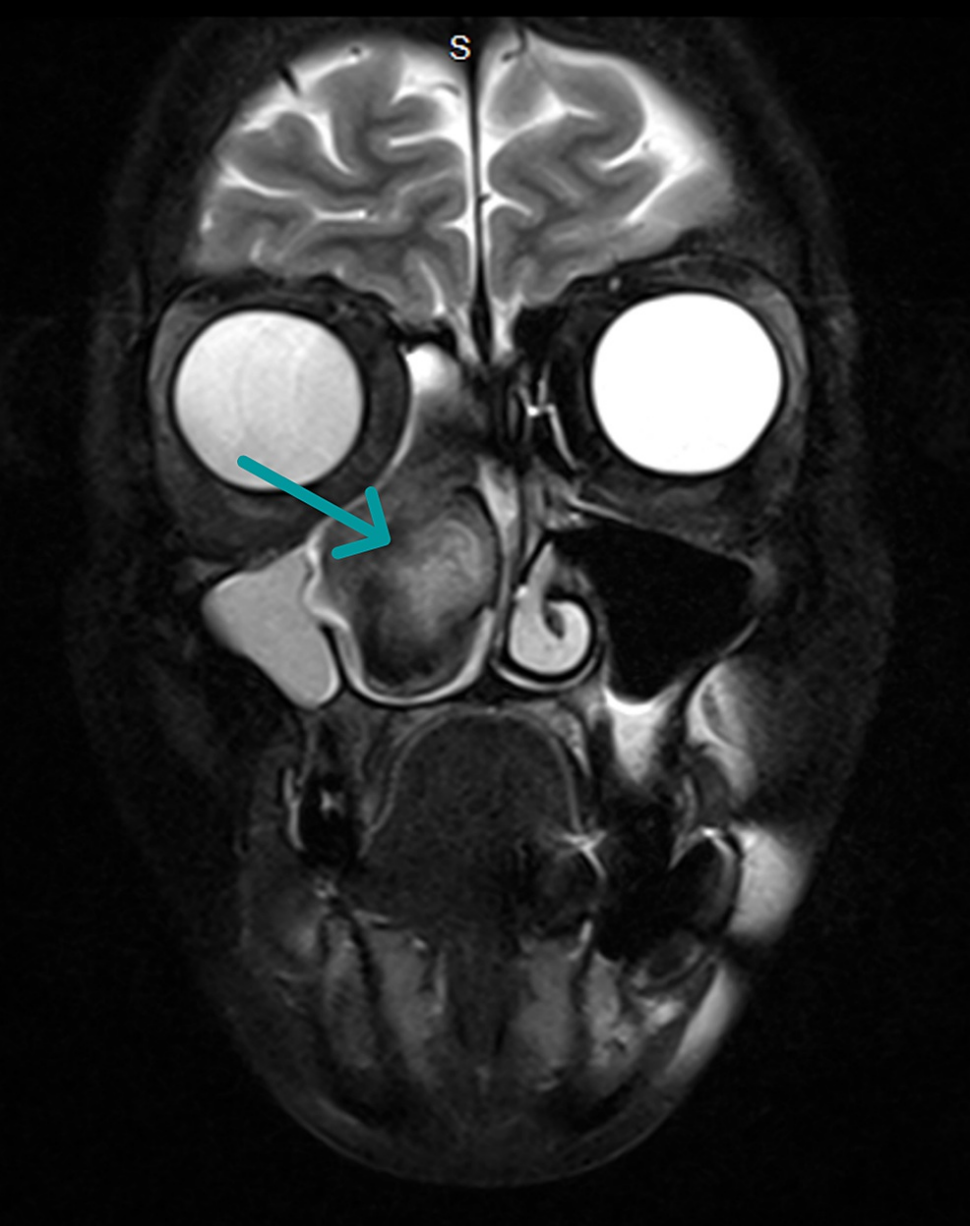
Brain and neck MRI The T2 coronal image shows a right maxillary sinus mass with heterogeneous signal intensity.
